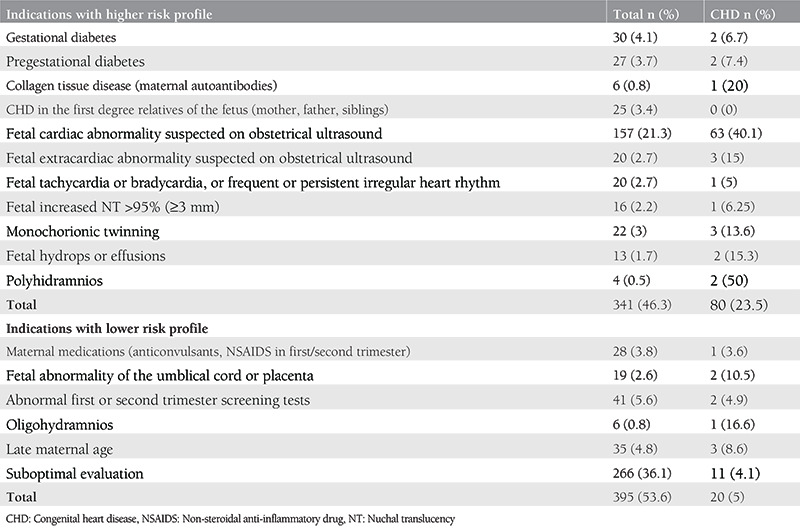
The distribution of the fetal echocardiography request reasons and the congenital cardiac malformation rates according to pregnancy risk profile*
